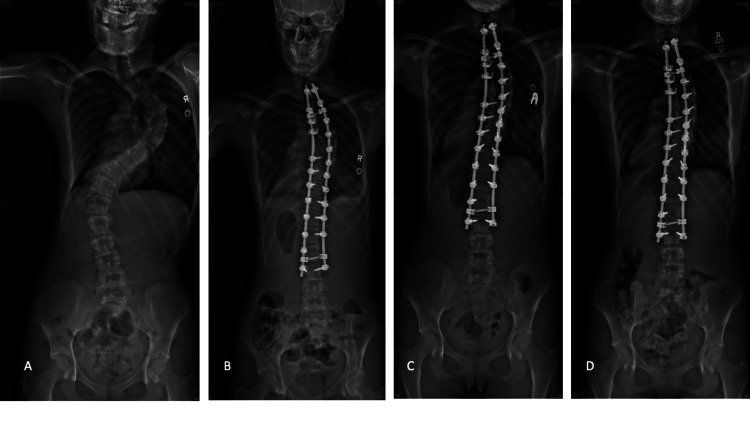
Patient diagnosed with adolescent idiopathic scoliosis with post-operative stable hook pullout. A patient diagnosed with adolescent idiopathic scoliosis with post-operative stable hook pullout: (A) pre-operative AP radiograph; (B) immediate post-operative AP radiograph; (C) AP radiograph showing a slipped proximal hook at the right T2; (D) no change in the position of the spine at two years following surgery.
